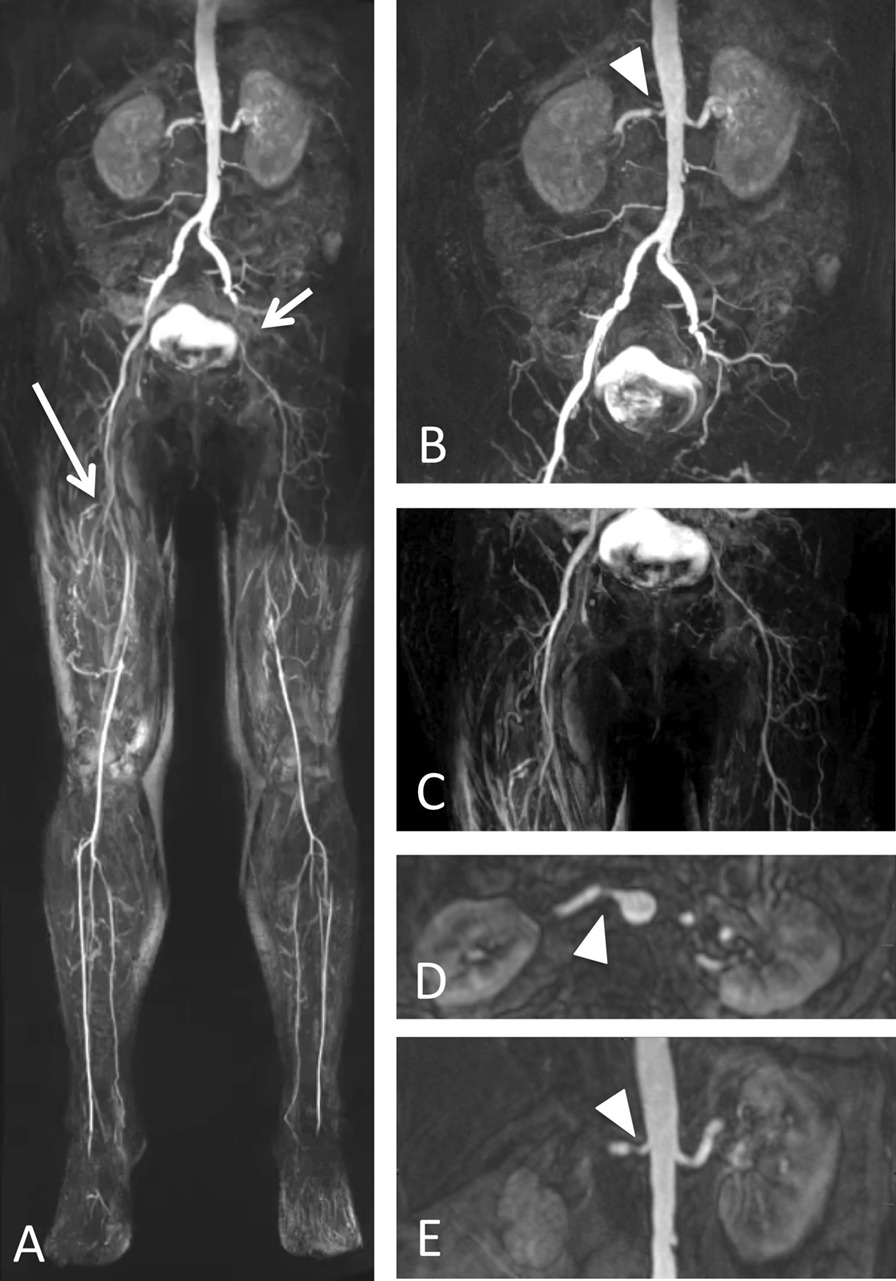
Run-off MR angiography in a 69-year old woman with known Stage IIb peripheral artery disease and hypertension. Subtracted images post contrast show occlusion of the proximal right superficial femoral artery (long arrow in a, magnified in c), the left external iliac artery and proximal left superficial femoral artery (short arrow in a, magnified in b). Subtracted image of the abdomen and 3D T1-weighted images post contrast of the visceral arteries demonstrate a stenosis of the right renal artery (arrowhead in b, d and e), which was classified as grade 3
